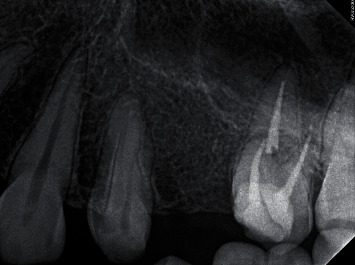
Postoperative intraoral periapical radiograph of the maxillary left first molar after a one-year follow-up period of retreatment: the radiolucent region of the tooth was healing.
